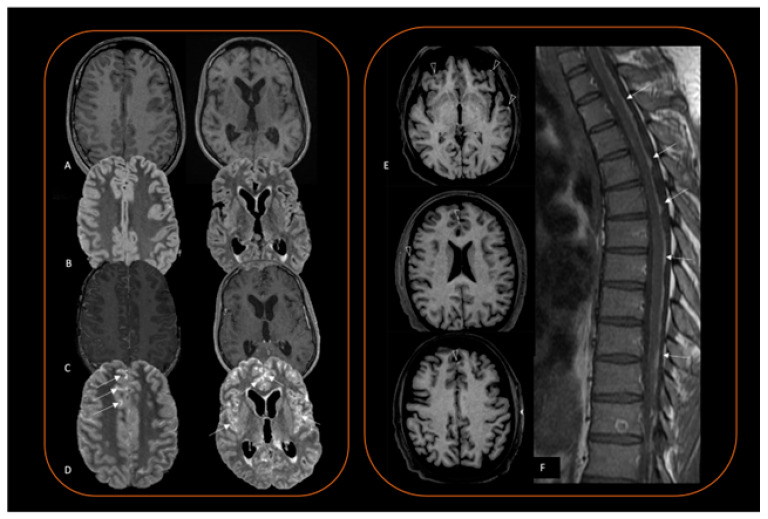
(A–D) A case of diffuse meningeal melanomatosis in a 14-year-old boy. In the first and second column, (A) 3D T1w sequence, (B) 3D FLAIR, (C) 3D T1w after contrast injection, (D) 3D FLAIR after contrast injection. This case displays the ability of delayed 3D FLAIR sequence to show leptomeningeal disease better than T1w post-contrast sequence (some examples of this increased visibility are marked with arrows). (E,F) Another case of diffuse meningeal melanomatosis (case courtesy of Darío Herrán de la Gala). (E) 3D T1w before contrast injection showing small focal areas of spontaneous T1 hyperintensity (arrowheads). (F) sagittal T1w sequence showing an extensive medullary involvement of the disease.
